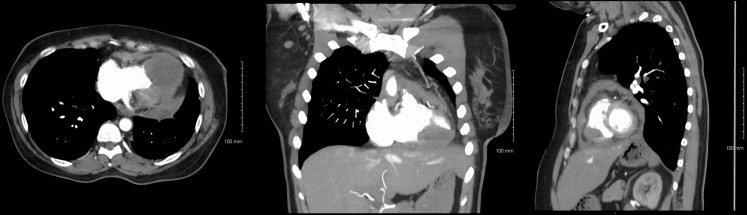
Axial (left), coronal (center), and sagittal (right) CT with contrast images showing intracardiac metastasis.
